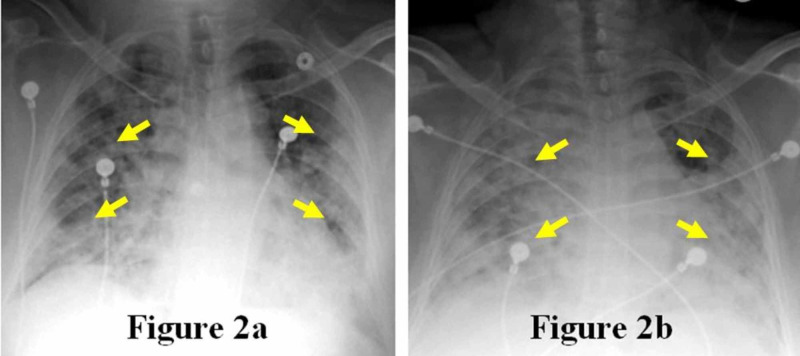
Initial and Subsequent Chest Radiographs Multifocal pneumonitis (arrows) on the initial radiograph (Figure 2a) and disease progression on the subsequent radiograph (Figure 2b).
